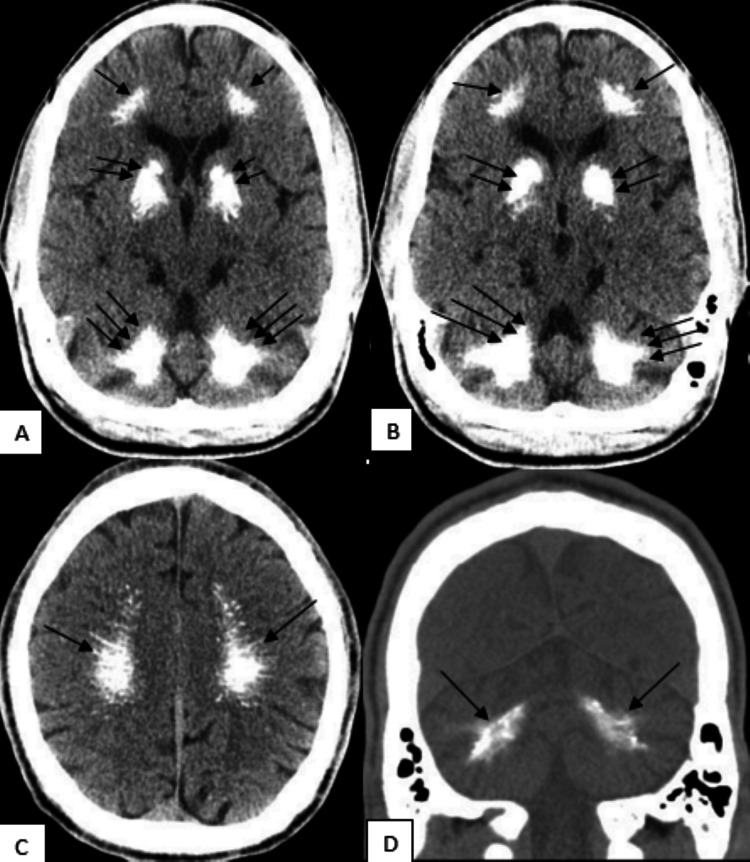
Non-contrast-enhanced CT of the head in axial planes (A-C) and a coronal plane (D) demonstrates large, diffuse, non-punctate calcifications bilaterally A-B: Axial plane CT. Single arrows demonstrate frontal cerebral white matter calcifications. Double arrows demonstrate basal ganglia calcifications, particularly in the globus pallidus region of the basal ganglia. Triple arrows demonstrate cerebellar dentate nuclei calcifications. C: Axial plane CT. Single arrows demonstrate cerebral centrum semiovale calcifications. D: Coronal plane CT. Single arrows demonstrate cerebellar dentate nuclei calcifications. CT: computed tomography
